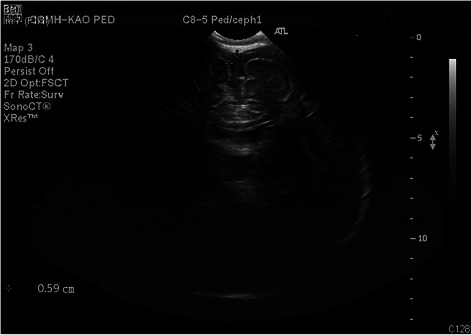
Brain ultrasound. Follow-up brain ultrasound 2 weeks after IVIG administration showing mild subdural fluid collection(0.59 cm)
